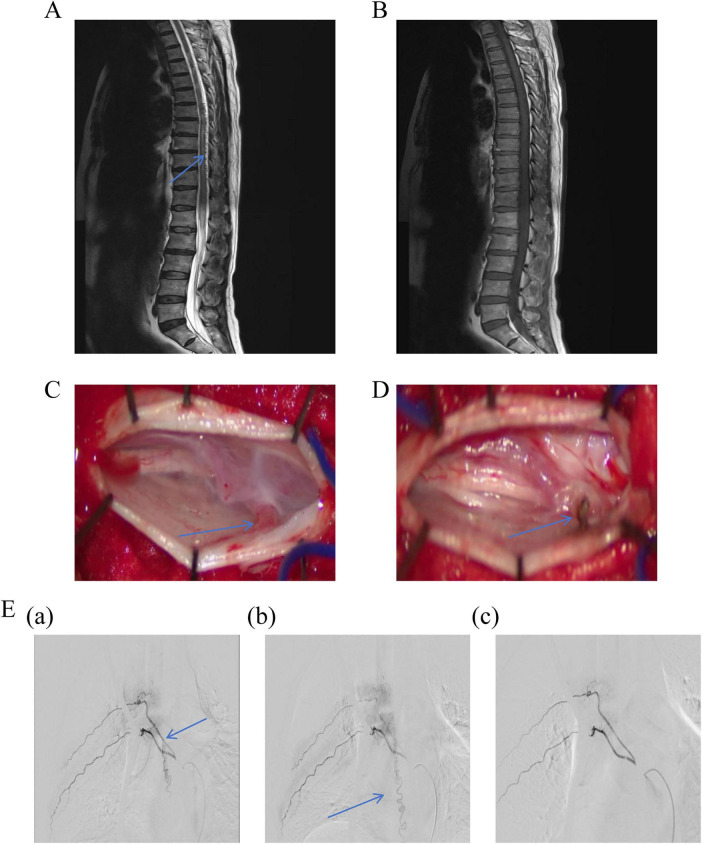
Radiological and intraoperative findings of spinal dural arteriovenous fistula (SDAVF). (A) Preoperative sagittal T2-weighted MRI showing longitudinal intramedullary T2 hyperintensity in the thoracic spinal cord and suspected dorsal serpiginous perimedullary flow voids (arrow), suggestive of venous congestive myelopathy. (B) Gadolinium-enhanced sagittal T1-weighted MRI of the thoracic spine, obtained as part of the preoperative evaluation. (C) Intraoperative view showing dilated and arterialized draining veins on the dorsal surface of the spinal cord (arrow). (D) Intraoperative view after disconnection of the fistula, demonstrating collapse of the abnormal draining vein (arrow). (E) Digital subtraction angiography (DSA): (a) Preoperative angiography revealing abnormal arteriovenous shunting at the right T5 level (arrow); (b) early venous drainage through dilated veins (arrow); (c) postoperative angiography confirming complete obliteration of the fistula.
